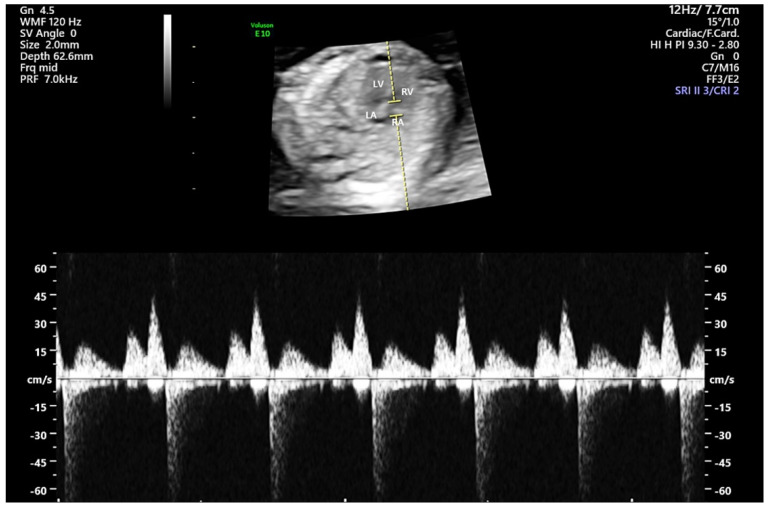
Pulse Doppler trace of fetal mild tricuspid regurgitation. LV, left ventricle; RV, right ventricle; LA, left atrium; RA, right atrium.
